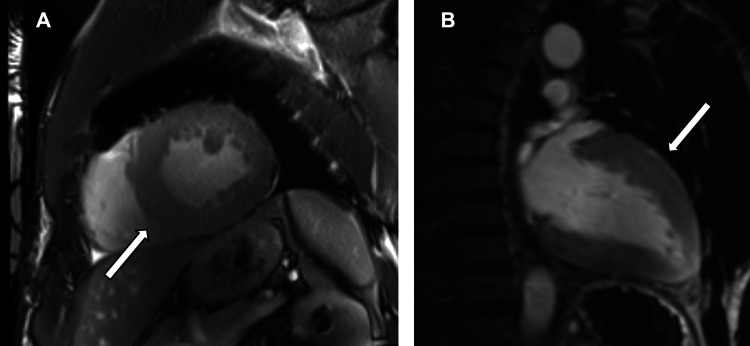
MRI cardiovascular images showing marked concentric left ventricle hypertrophy (arrows) in the (A) two-chamber (2CH) and (B) three-chamber (3CH) planes.
